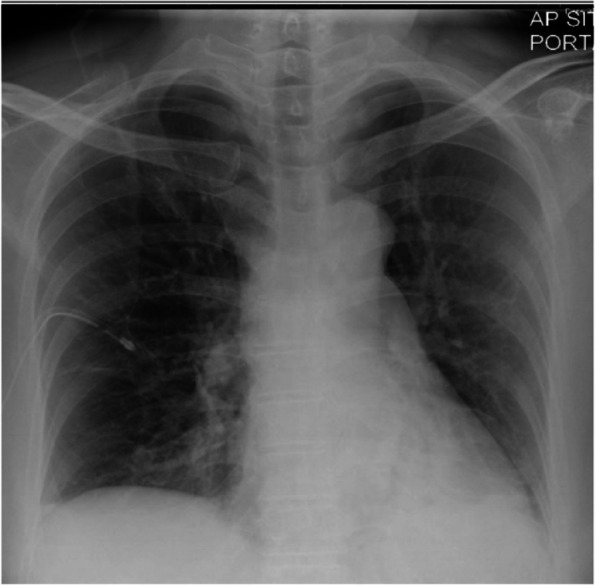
Chest X-ray after insertion of Seldinger chest drain showing reduced small right pneumothorax with a width of about 1.5cm at the apex. A small left apical pneumothorax is still present
